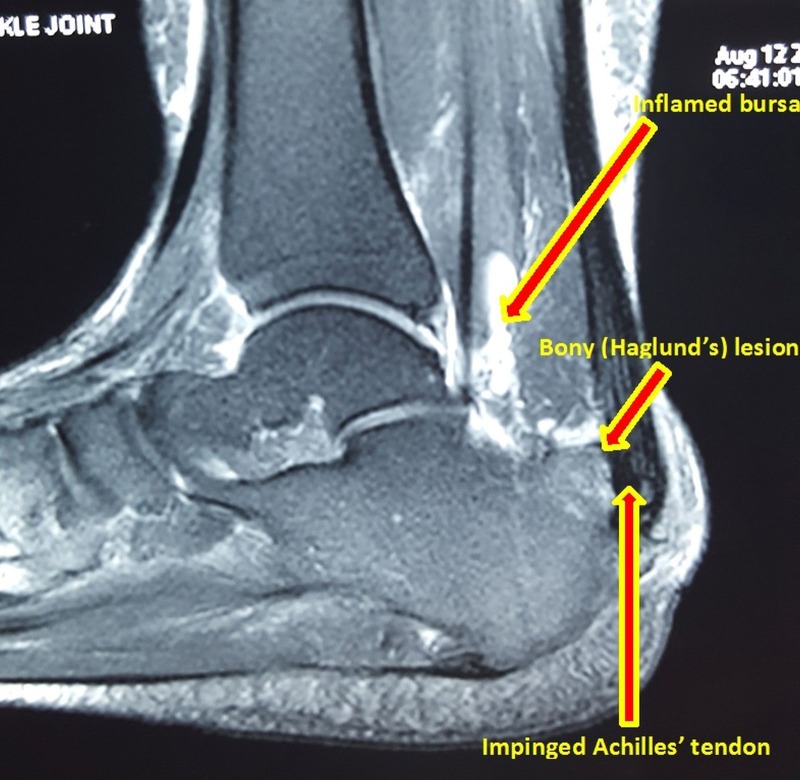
MRI image of ankle and foot showing posterosuperior bony spurring of calcaneus, retrocalcaneal bursitis, and impingement of Achilles tendon
